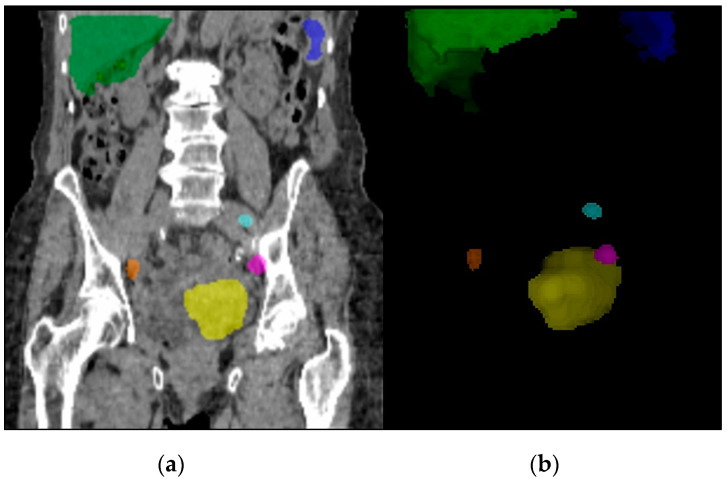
Segmented volume-of-interests (VOIs) for SPECT/CT quantification in an endometrial cancer (EC) patient. The case study shows VOIs for liver (green), spleen (blue), injection site (yellow), and three bilateral iliac SLNs (other colors) in coronal low dose CT (a) and in tridimensional representation of the regions (b).
